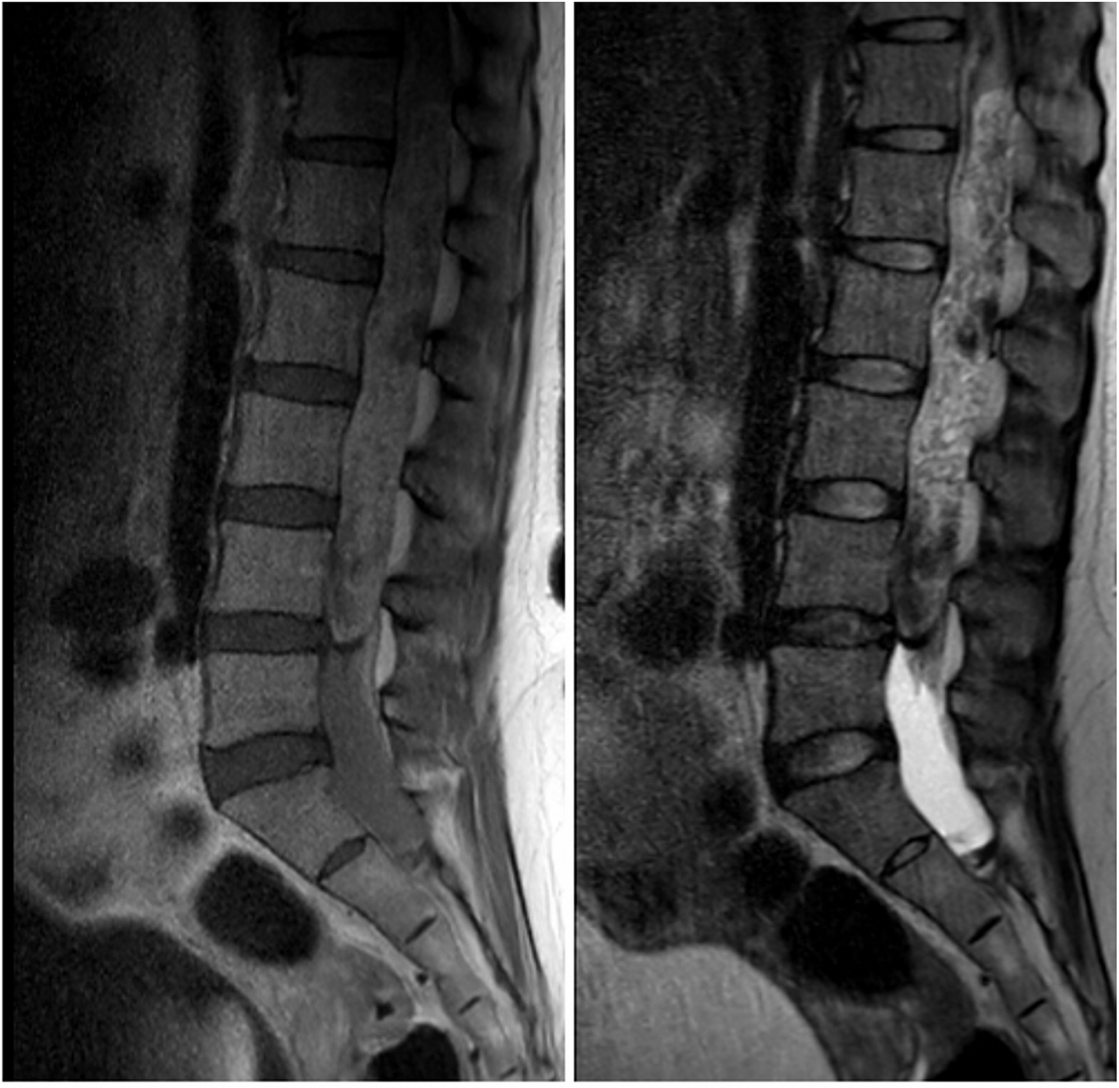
Initial lumbar magnetic resonance image (MRI) scan. T1-weighted MRI (left). T2-weighted MRI (right). There are extensive T2 signal hypointensities within the mass, representing small vascular structures and blood products.
